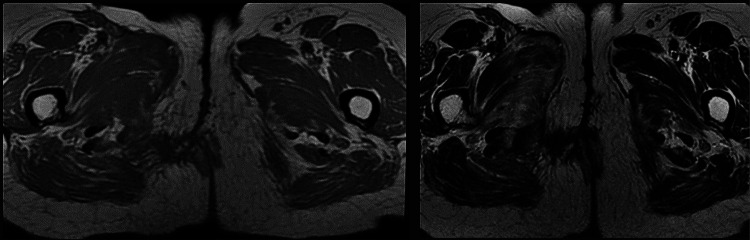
Follow-up MRI performed after completion of radiation therapy showed no local recurrence.
